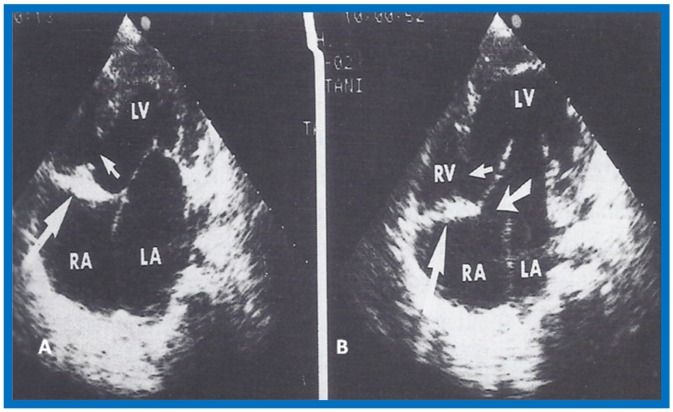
Apical four-chamber view pictures of another infant with tricuspid atresia (large arrows in A,B) with ostium primum atrial septal defect (slanted arrow in B). Note small right ventricle (RV) and a ventricular septal defect (small arrows in A and B). LA, left atrium; LV, left ventricle; RA, right atrium. Reproduced from Covitz W., Rao P.S. [36].
